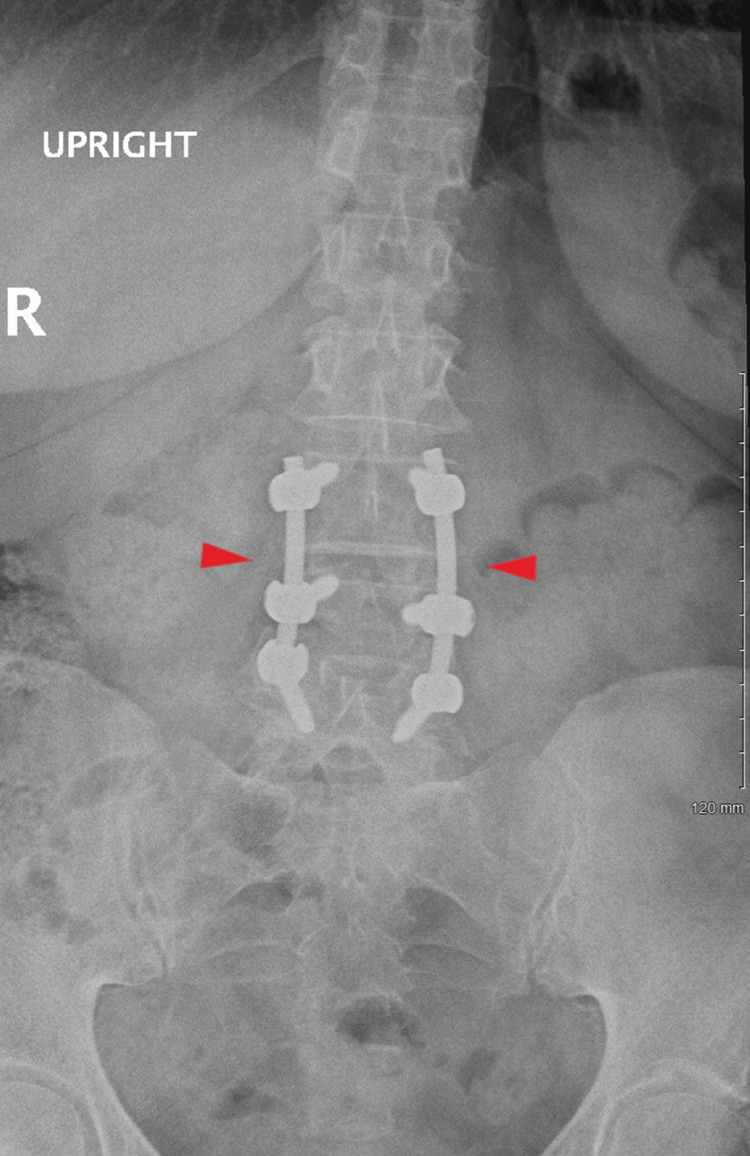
Lumbar X-ray showing postoperative changes of the posterior spinal fixation and decompression from L3-L5 with good alignment and good placement of hardware (Age: 56 years).
